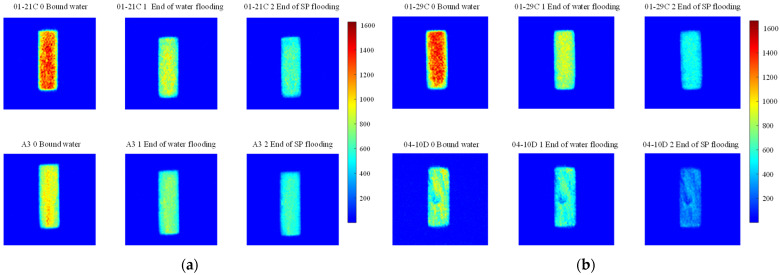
MRI dynamic monitoring map of core flooding (along flooding direction): (a) Combinations 11 and (b) 12.
